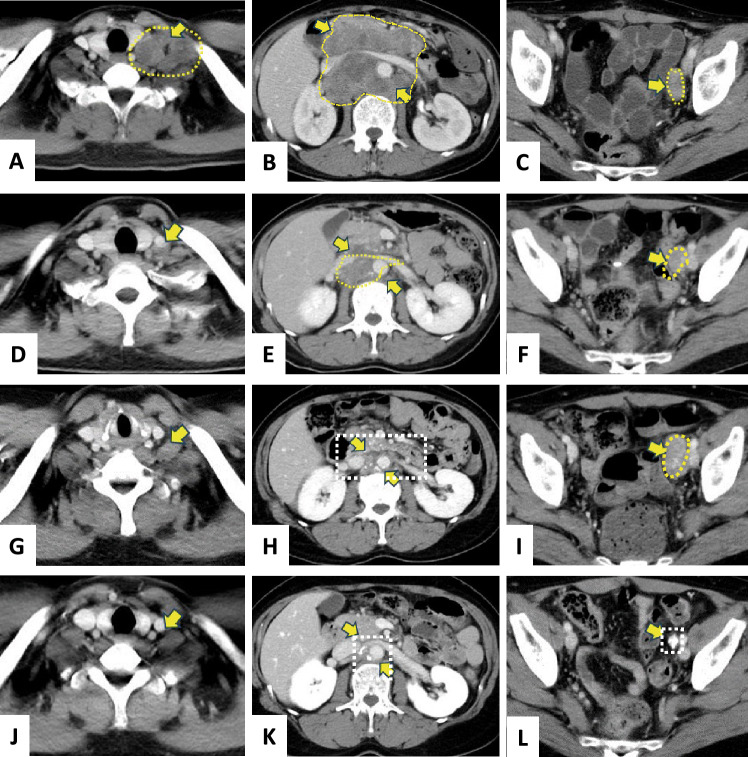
CT scans of target lesions A. LSLN metastasis in May 2020. B. PAN metastasis in May 2020. C. LEIALN metastasis in May 2020. D. LSLN metastasis in August 2020. E. PAN metastasis in August 2020. F. PAN metastasis in August 2020. G. LSLN metastasis in January 2021. H. PAN metastasis in January 2021. I. LEIALN metastasis in January 2021. J. LSLN metastasis in May 2024. K. PAN metastasis in May 2024. L. LEIALN metastasis in May 2024. The yellow arrows and dots mark the lesion area
